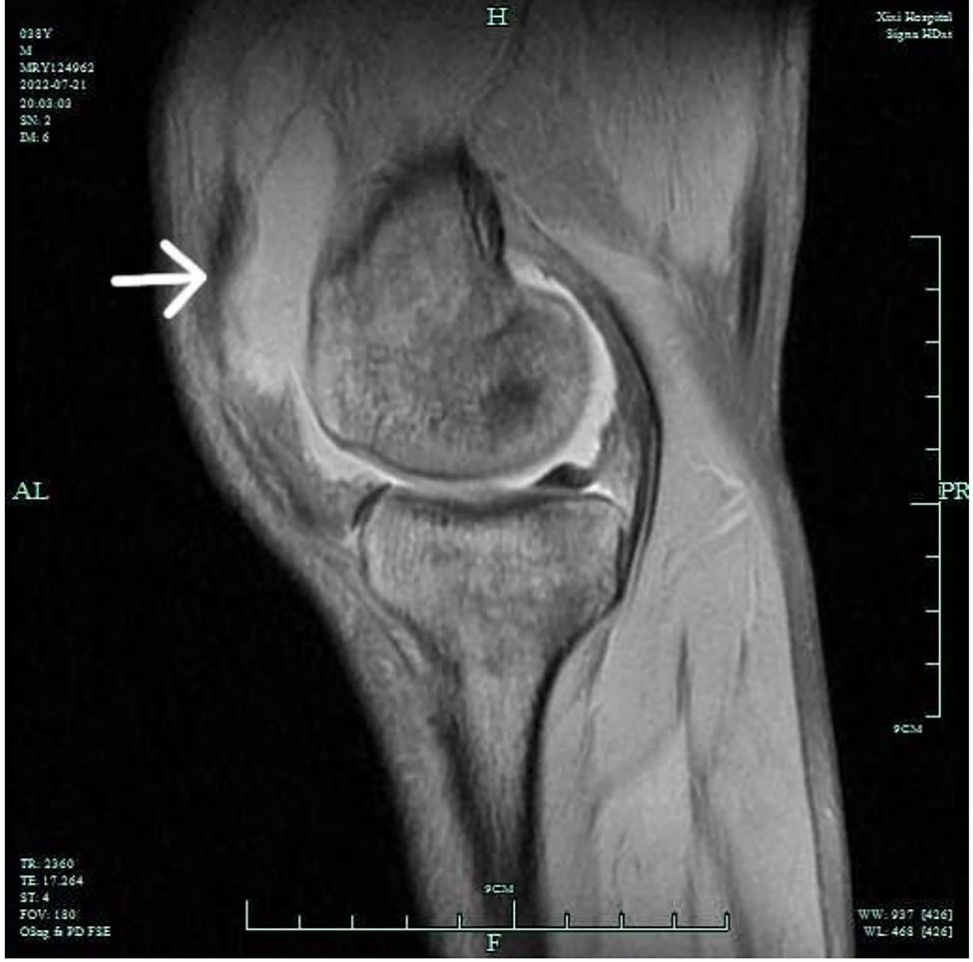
MRI of the left knee joint revealed numerous patchy long T1 and long T2 signal lesions in the knee bones, with indistinct edges. Abundant fluid signals were noted in the joint space and upper abdominal bursae, accompanied by swelling of the surrounding muscles and soft tissues
